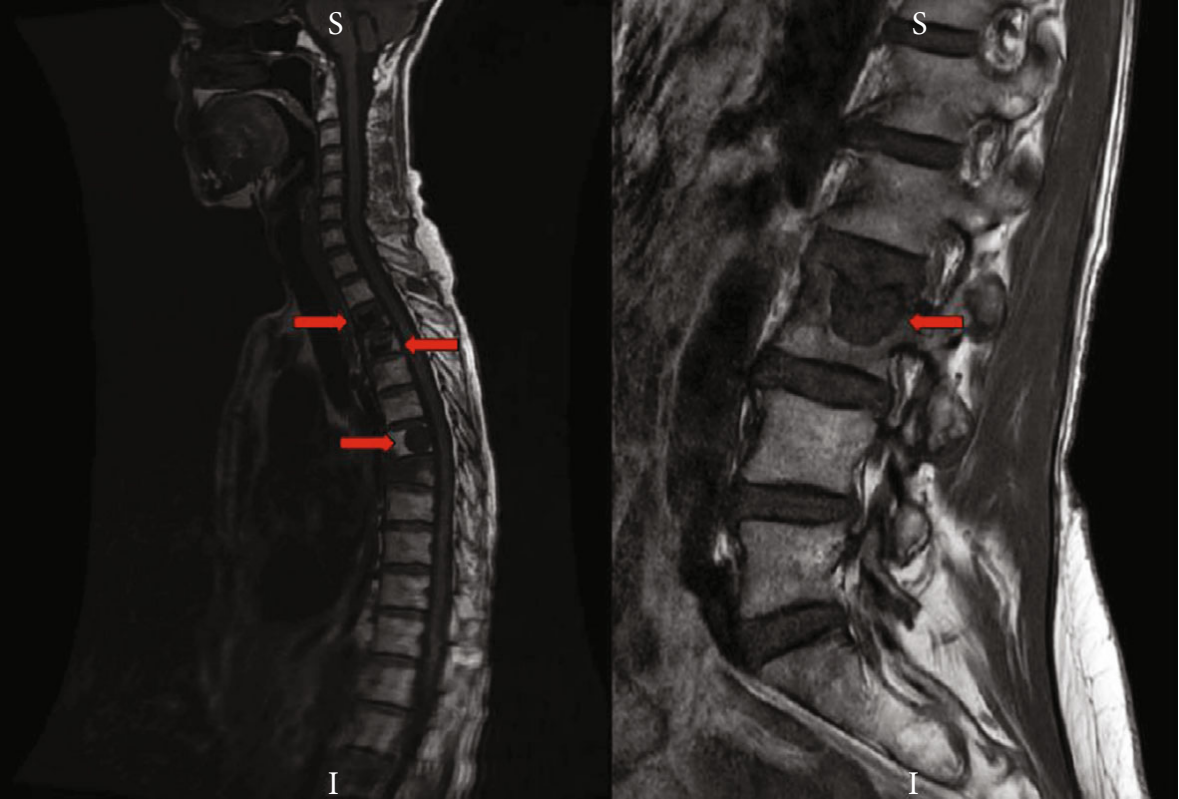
MRI of the thoracic and lumbar spine showing multiple osseous lesions.
